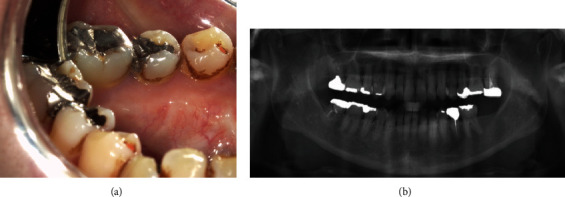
Follow-up oral examination 2 years after the surgery. (a) Follow-up observation revealed complete soft tissue healing with no signs of refractory osteonecrosis and exposed bone. (b) Panoramic radiography was also clear.
